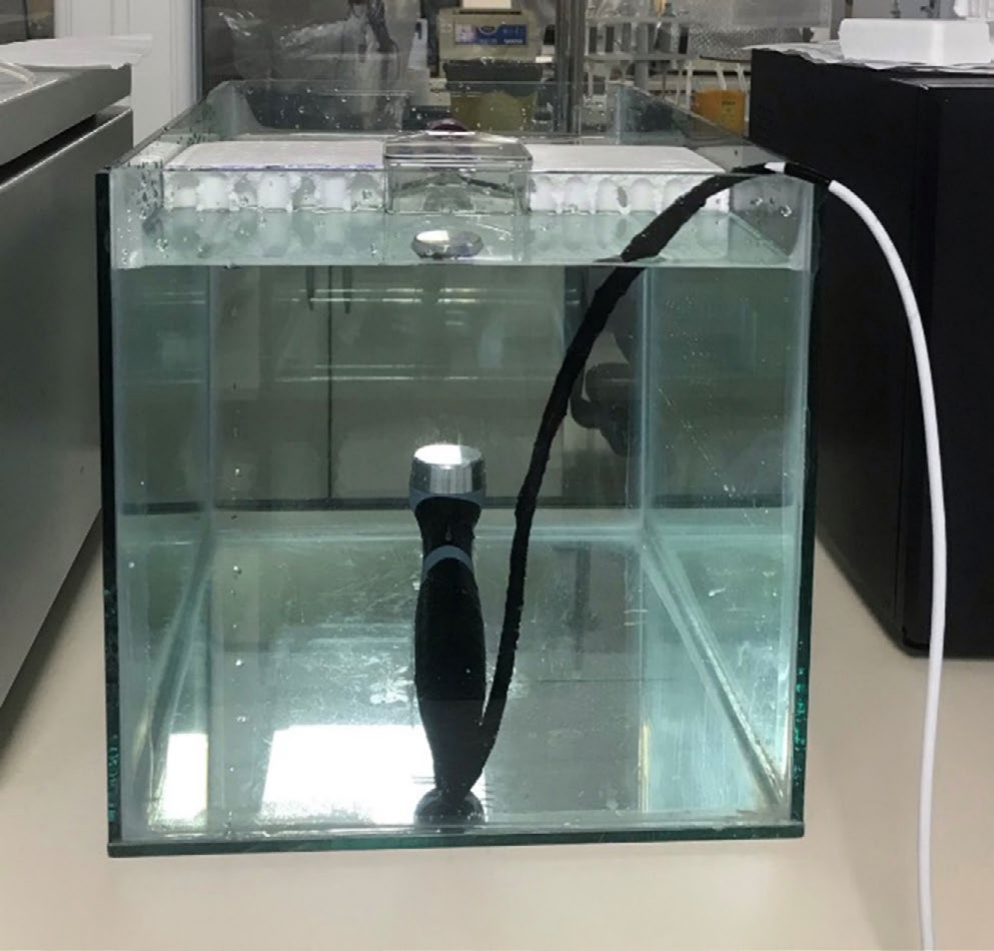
Administration of ultrasound therapy.
